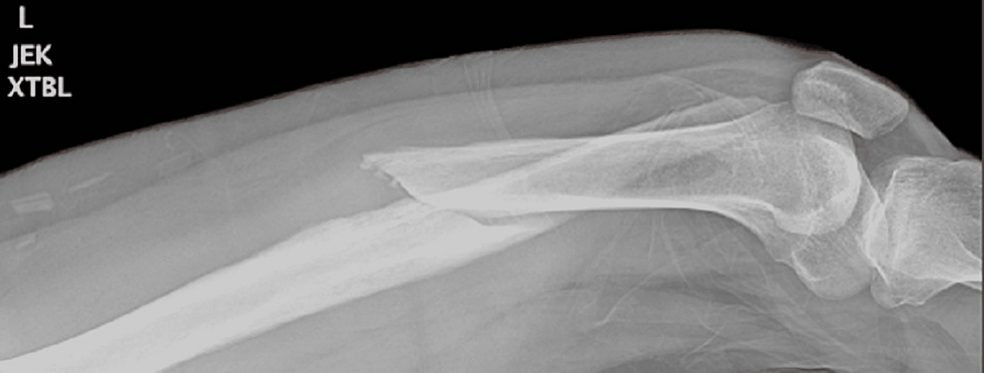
Femur x-ray showing the distal displaced femoral fracture (view 1)
